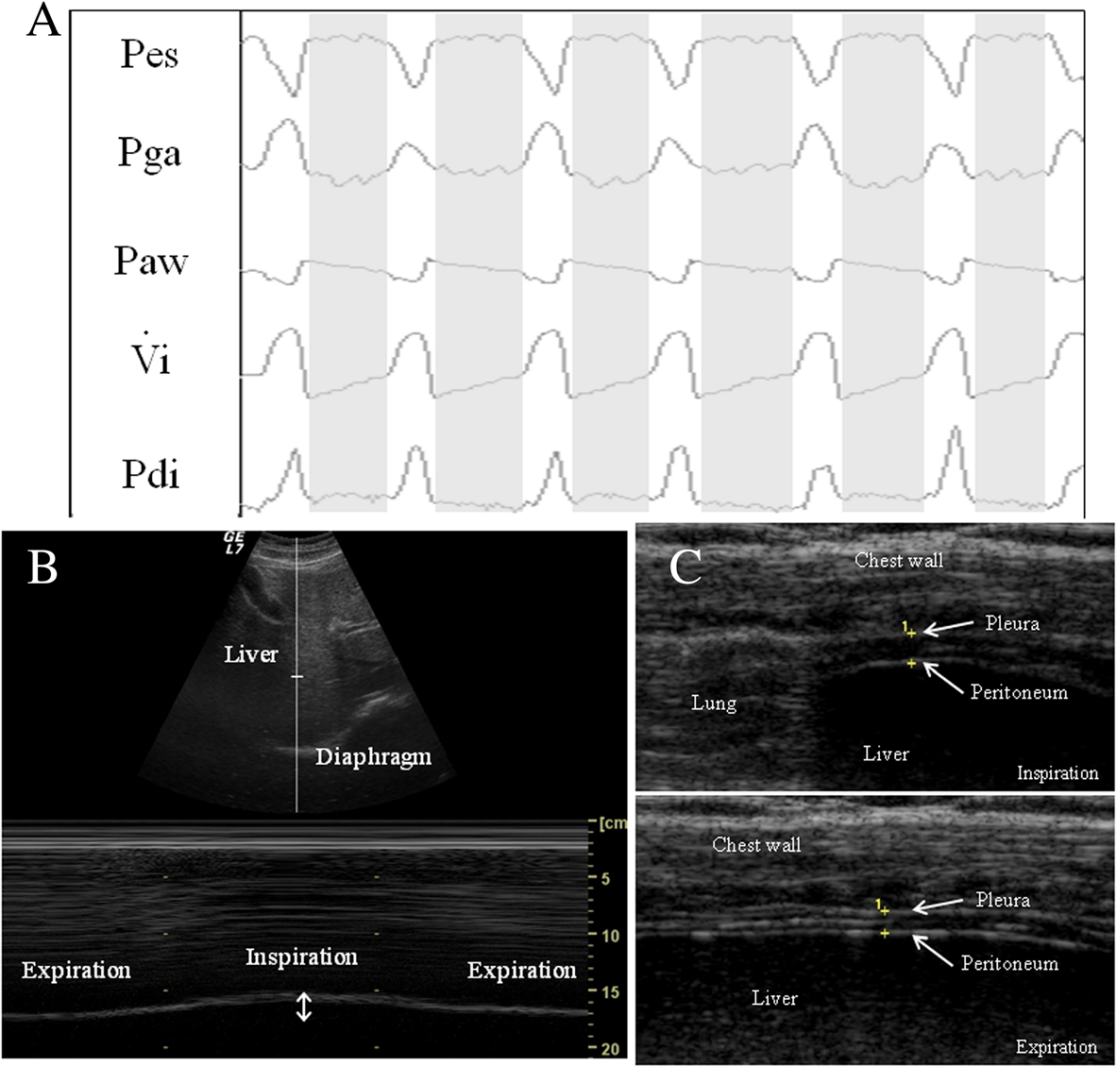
Example of respiratory tracing and ultrasonographic measurements during the 0 cmH
